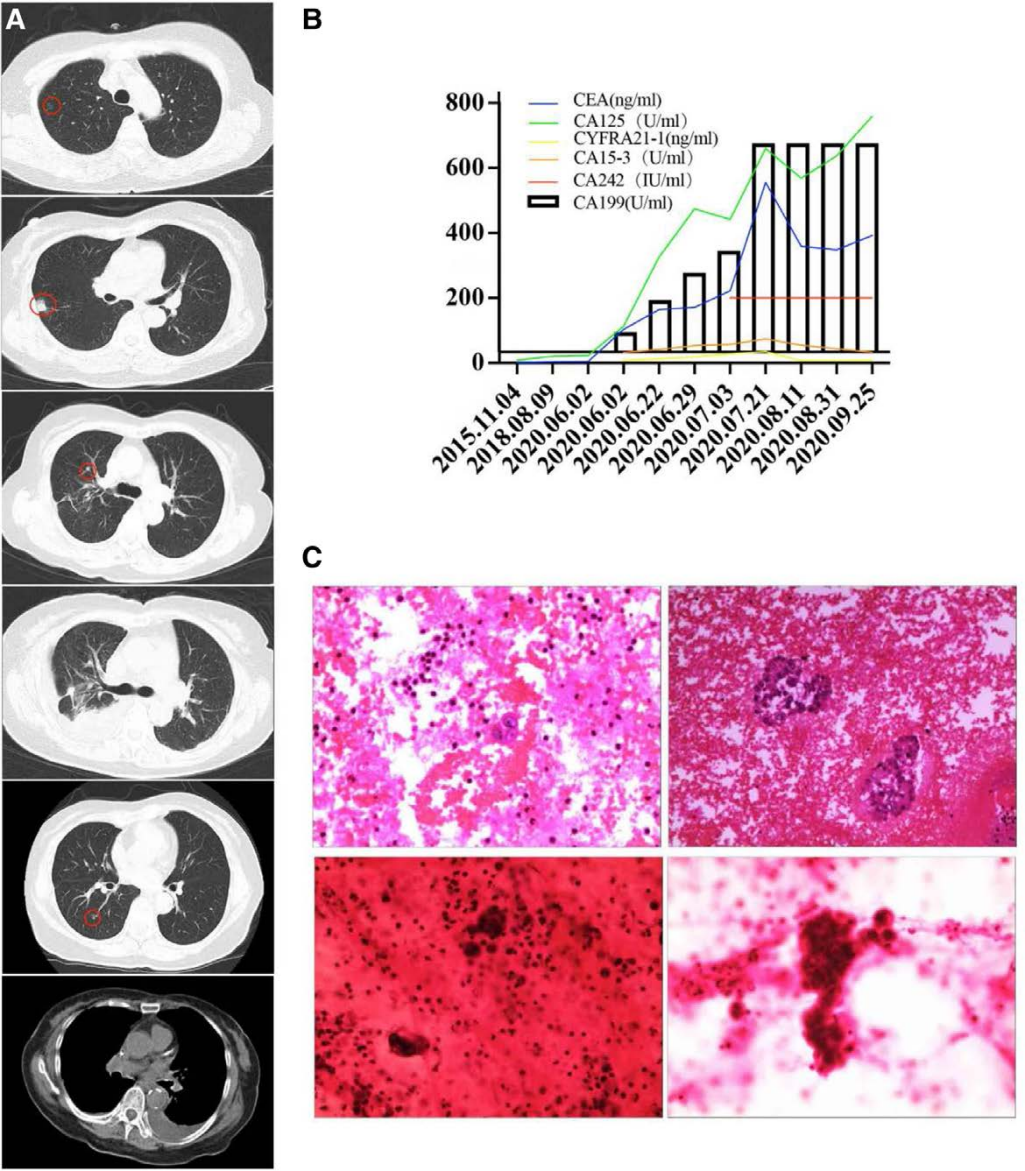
(A) The chest CT outcomes during the whole treatment. Ground-glass nodules in the upper lobe of the right lung (diameter 5.7 mm) and subpleural nodules in the upper lobe of the right lung (diameter 12.8 mm) were first detected by chest CT on July 15, 2015. A right upper lobe nodule (approximate diameter 7.9 mm) with a short marginal burr was observed 9 months after surgery. Pleural effusion and pericardial effusion were detected on July 1, 2017. The 7-mm diameter ground glass nodules were observed 24 months after initiation of crizotinib treatment. There was a recurrence of pleural effusion and pericardial effusion on October 8, 2020. (B) The trend of change in serum cancer markers. (C) Adenocarcinoma cells were found in pericardial effusion and pericardial effusion wax blocks on June 15, 2017. Adenocarcinoma cells were found in bile drainage smears on July 7, 2020. CT = computed tomography.
